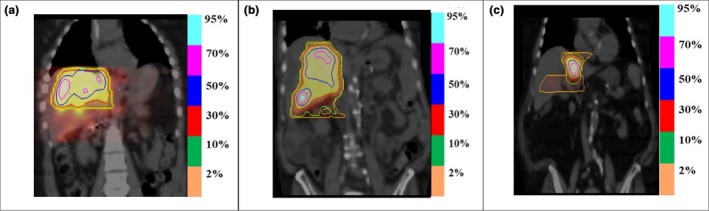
Coronal slice through single photon emission tomography (SPECT) dose map with isodose lines fused with computed tomography showing ^90^Y microsphere dose distribution for patients 6(a), 8(b), and 12(c).
